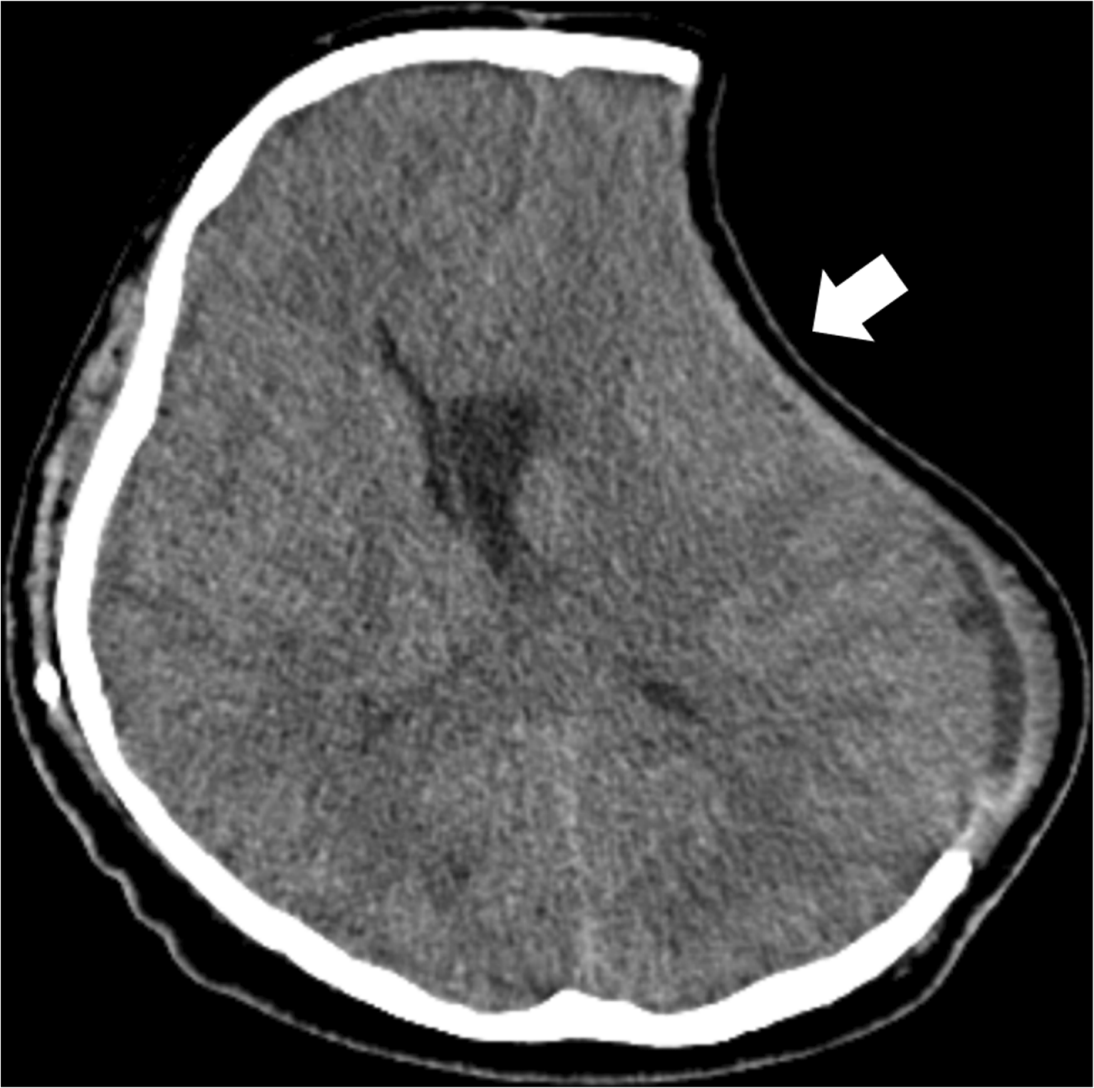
Sunken skin flap after DC. Axial CT scan showing a sunken skin flap (white arrow) after left-sided hemicraniectomy
